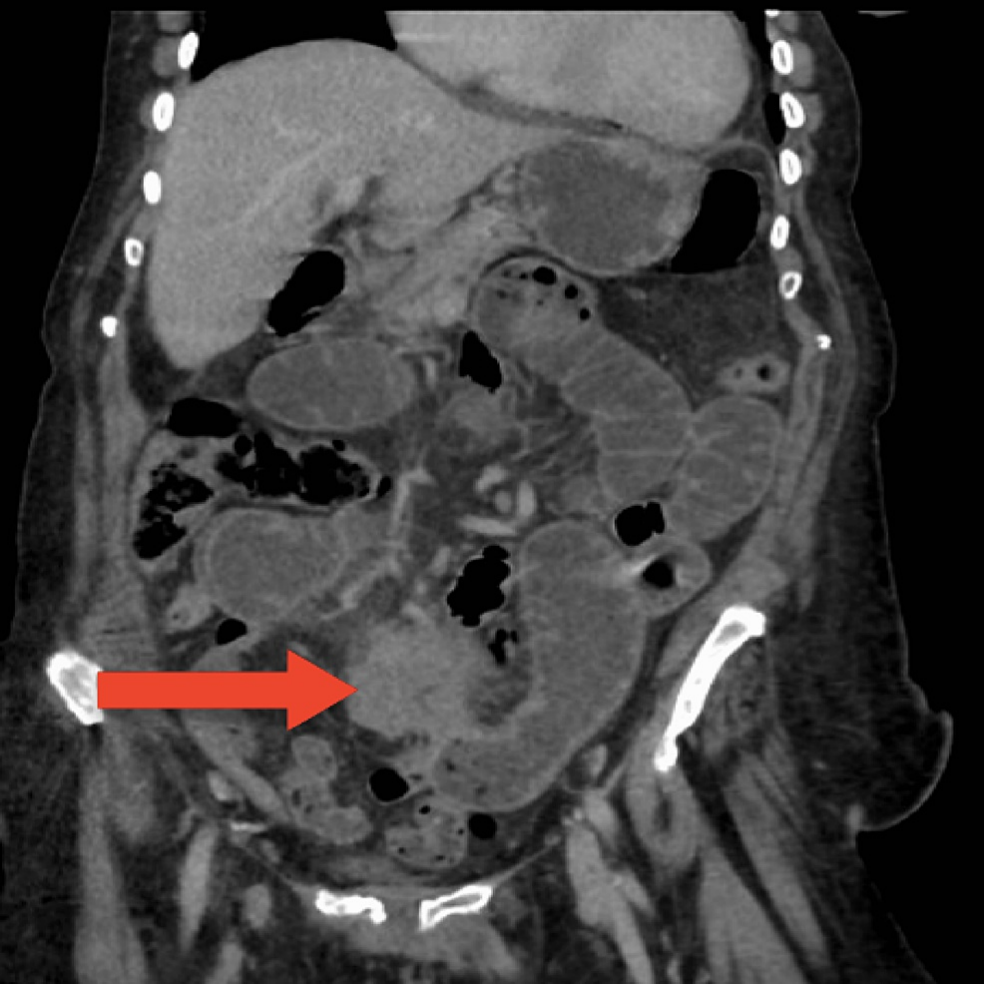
CT coronal view showing the mass (red arrow)
